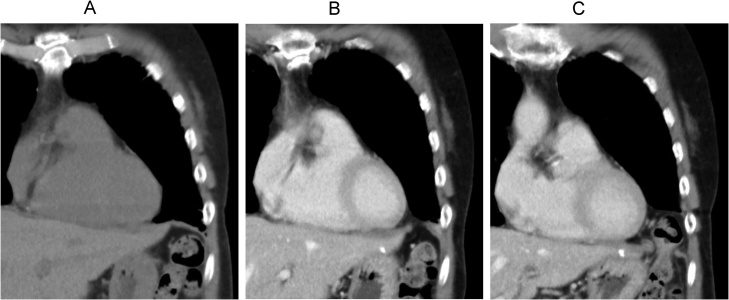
Coronal computed tomography images showing the left diaphragm after the first laparoscopy. (A) At 2 months, the diaphragm seemed intact. (B) At 6 months, a left diaphragmatic hernia was observed. (C) At 9 months, incarcerated splenic flexure of the colon was observed.
